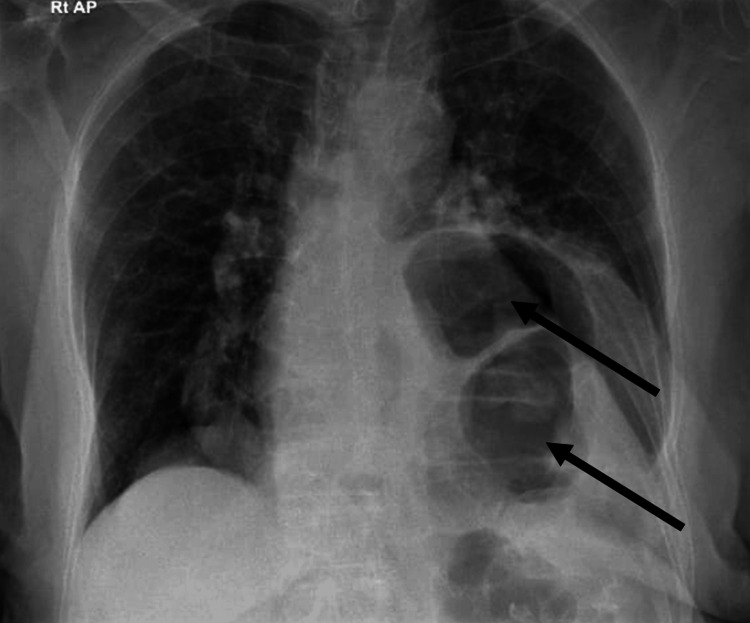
Posteroanterior Chest Radiograph Demonstrating Findings Consistent with Giant Hiatal Hernia. A large retrocardiac opacity is identified in the left hemithorax, containing gas-filled bowel loops representing herniated abdominal viscera (black arrows). Associated findings include leftward mediastinal shift and dorsal scoliosis convex to the right, reflecting the mass effect of the intrathoracic hernia on surrounding mediastinal structures. Reduced left lung volume secondary to compression by the herniated contents is also noted. These radiographic findings prompted further cross-sectional imaging to confirm the diagnosis.
